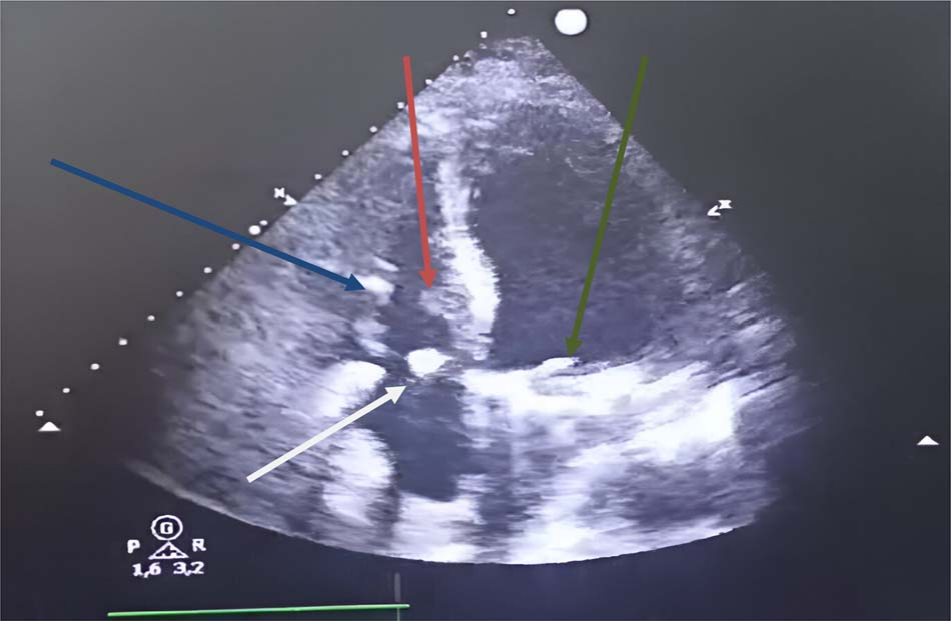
Postoperative transthoracic echocardiography, four chambers showed at 1 month. Green arrow: mechanical mitral prosthesis in place without stenosis and without detectable leakage. White arrow: tricuspid coaptation without detectable leakage, with slight excess fabric at the suture line on the anterior tricuspid leaflet. Blue arrow: inferior tricuspid papillary muscle. Red arrow: anterior tricuspid papillary muscle.
